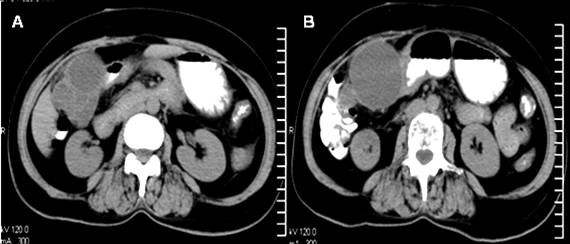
A computed tomography scan image showing the multilocular cyst with septations (a) and its proximity to the duodenum and colon (b).
